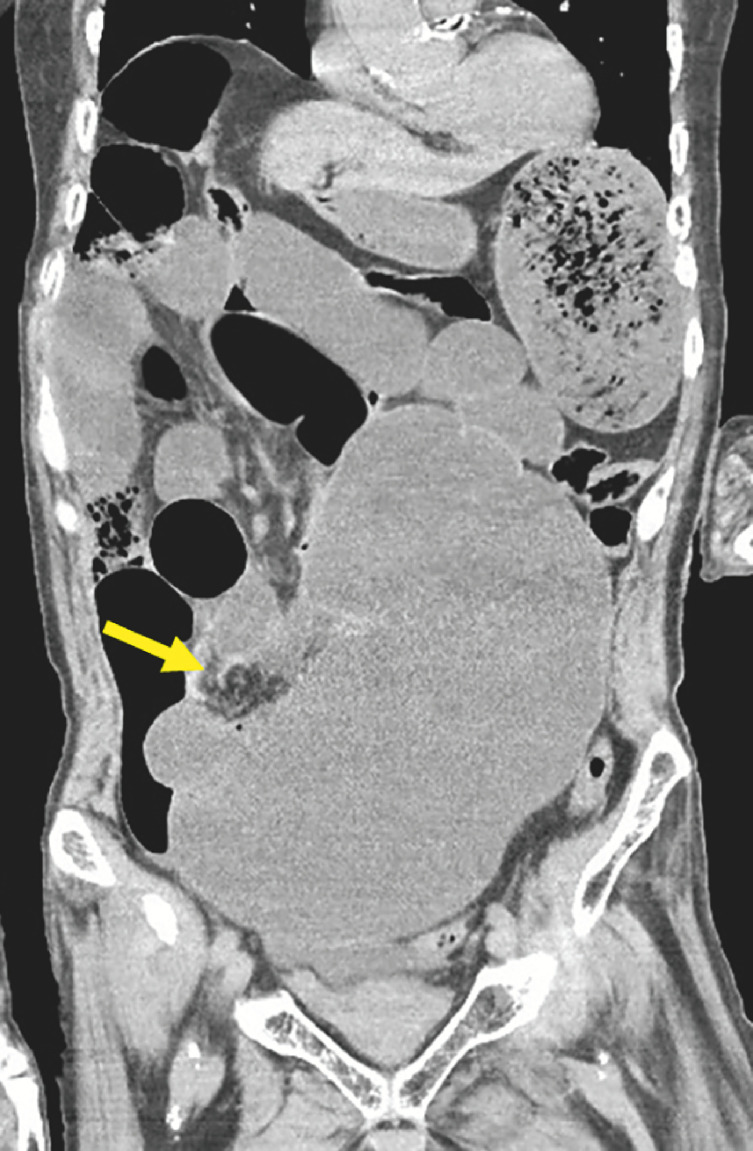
Computed tomography scan showing a grossly dilated and inverted cecum, along with mesenteric whirl signs (arrow).
